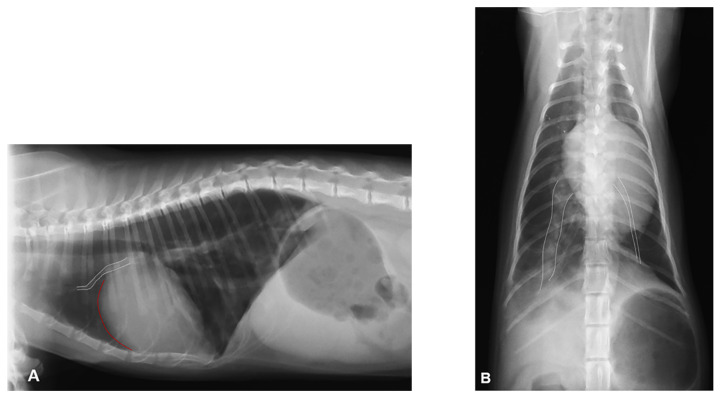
Thoracic radiography at presentation shoving an interstitial pulmonary pattern in the right caudal lung lobe: (A) Right lateral view showing cardiomegaly with increased convexity of the cranial cardiac border (red line) and prominent and tortuous right cranial pulmonary artery (white dotted lines). (B) Dorso-ventral view showing severe asymmetry of the caudal pulmonary arteries with severely enlarged and hypovascular right and left caudal pulmonary artery, respectively (white dotted lines).
